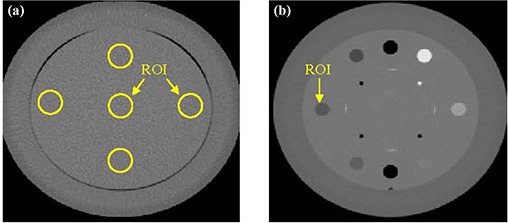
CBCT slices that represent: (a) the uniformity module with regions‐of‐interest (ROI) used to calculate average CT and standard deviation; (b) the linearity module.
